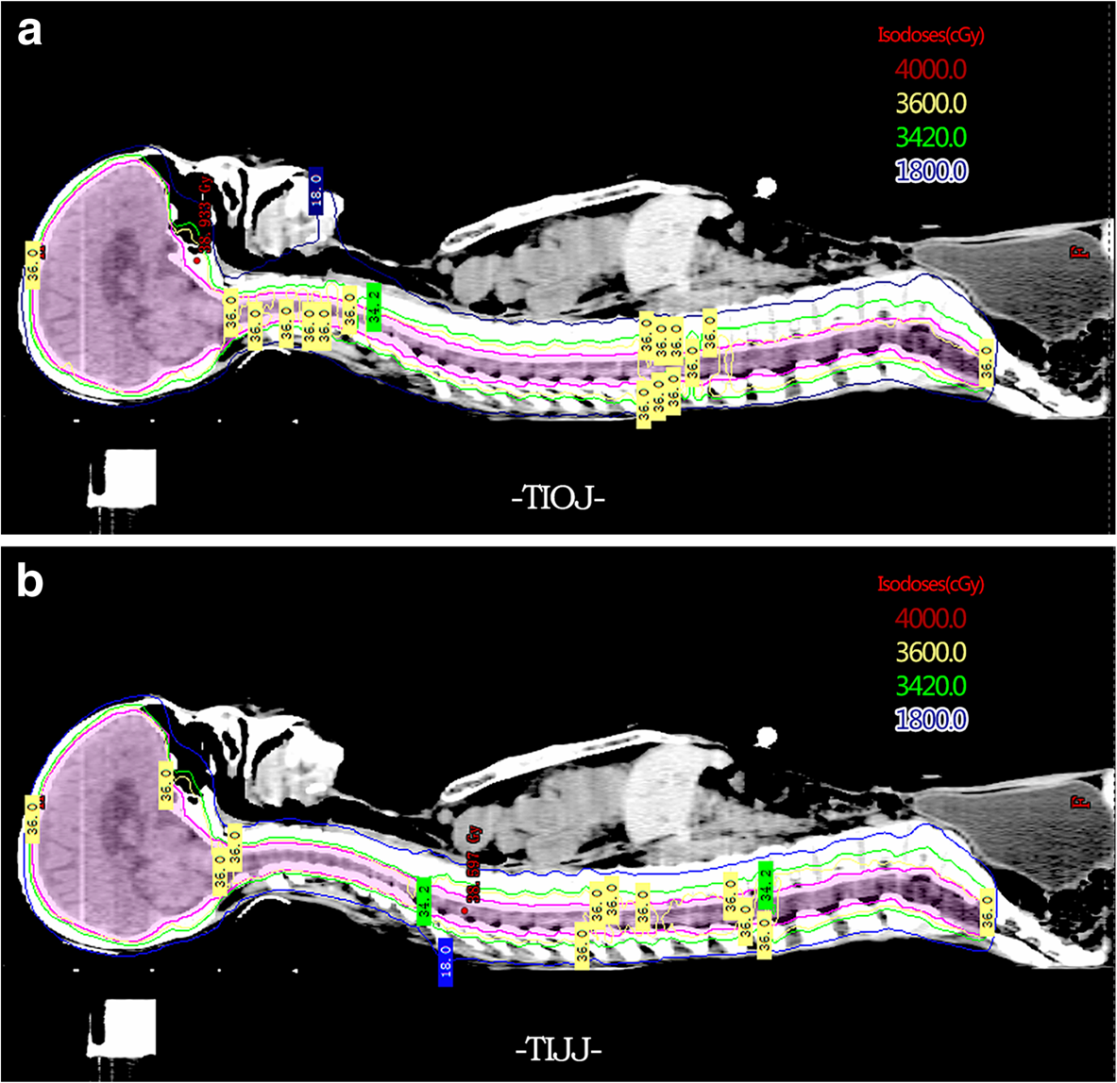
Isodose distribution of Patient 5 for TIOJ plan and TIJJ plan. Isodose distribution on a midline sagittal CT slice of Patient 5 for TIOJ plan (a) and TIJJ plan (b). Color legend indicates isodose lines, in centigrays. Planning target volume is shown in pink.
